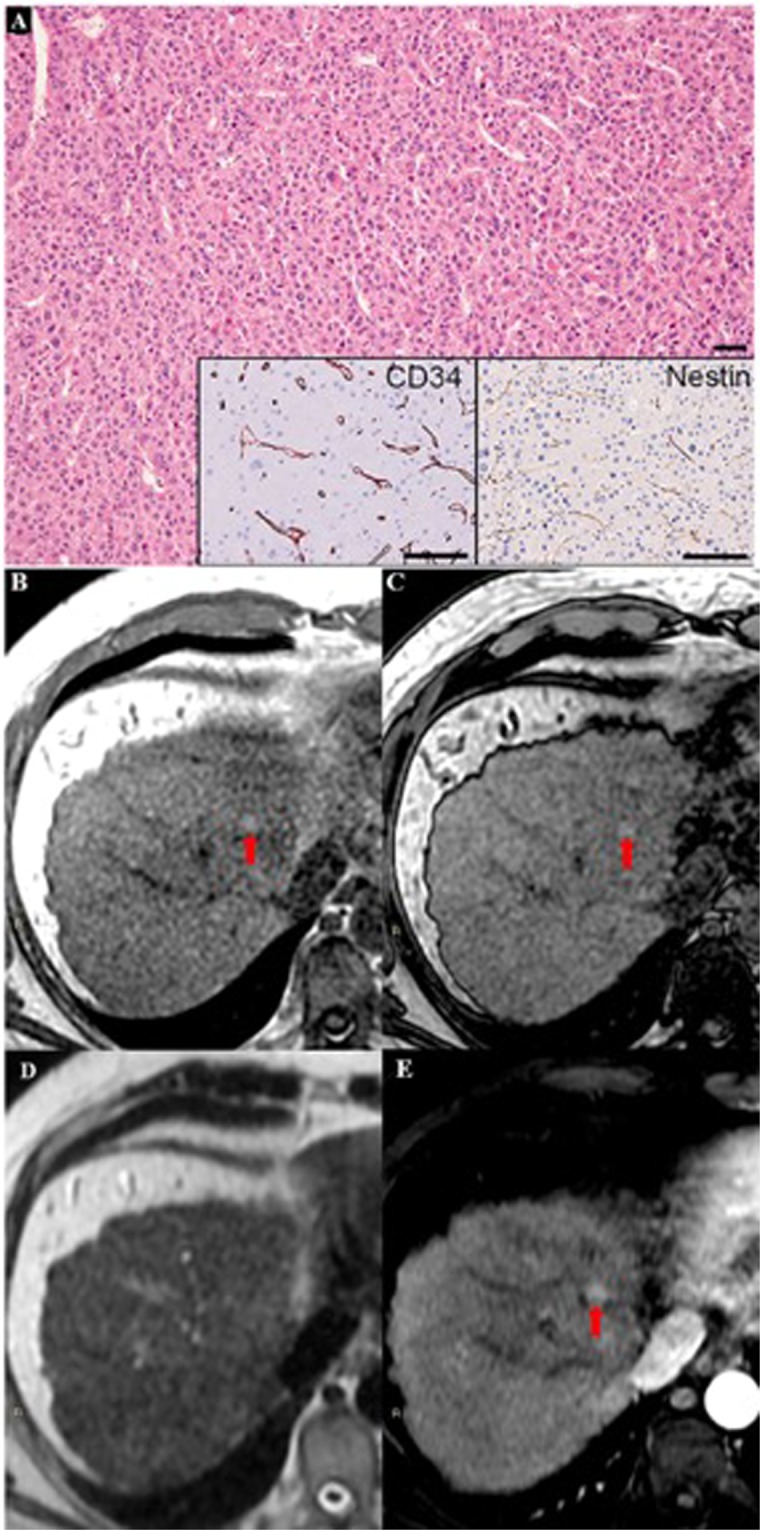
A case of HCC with morphovascular Pattern B, similar to the case in Fig. 1 in morphology, but with sinusoids positive for both CD34 and Nestin (A). In MRI, a clear nodule was identified in the liver segment IV, with hyperintensity in T1-weighted images in-phase (B) and out-phase (C), without hyperintensity in T2-weighted image (D) and with arterialization (E).
